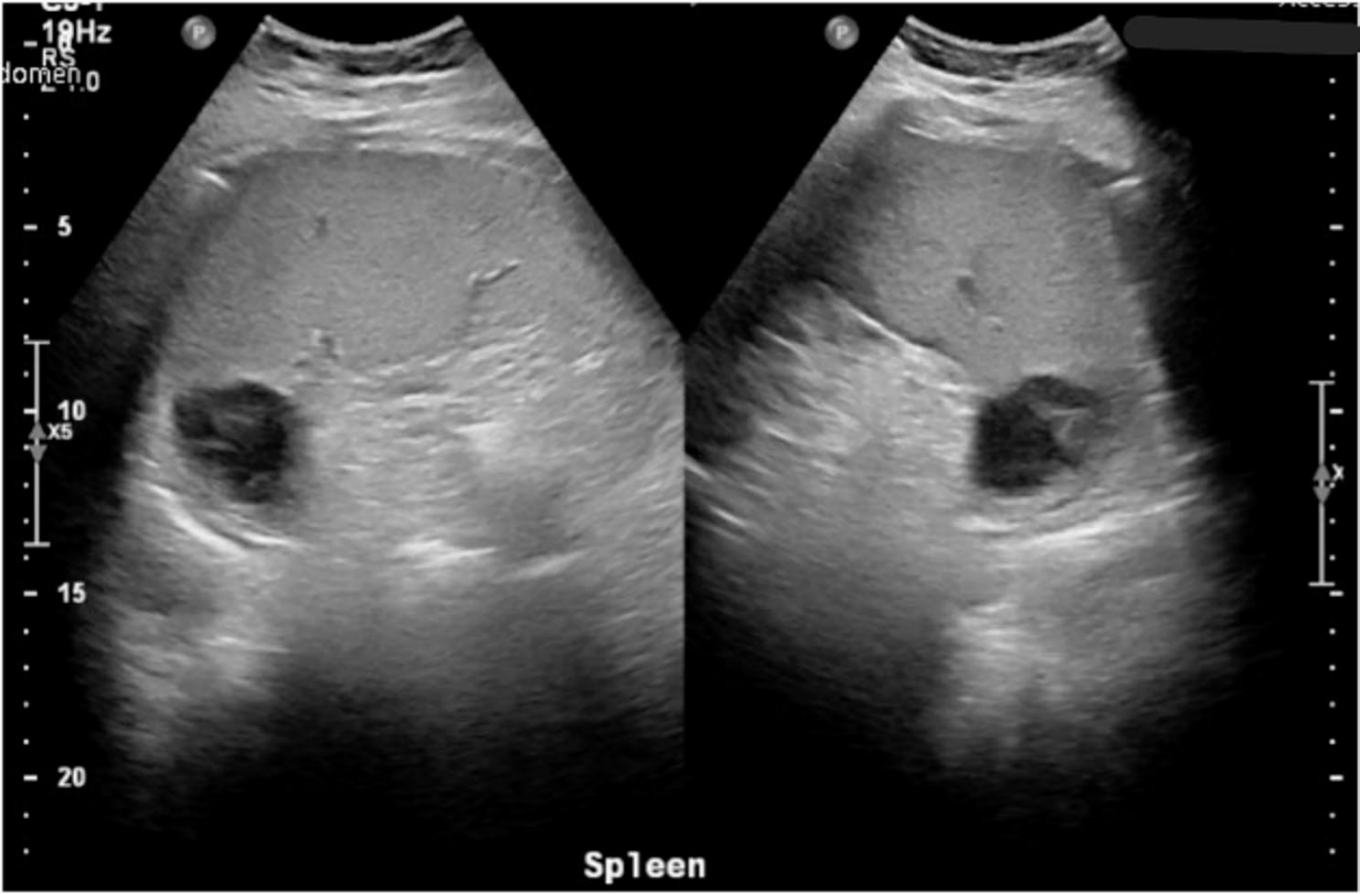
Abdominal ultrasound exam showing a 4 × 3 cm heterogenous hypoechoic structure in the superior splenorenal region
